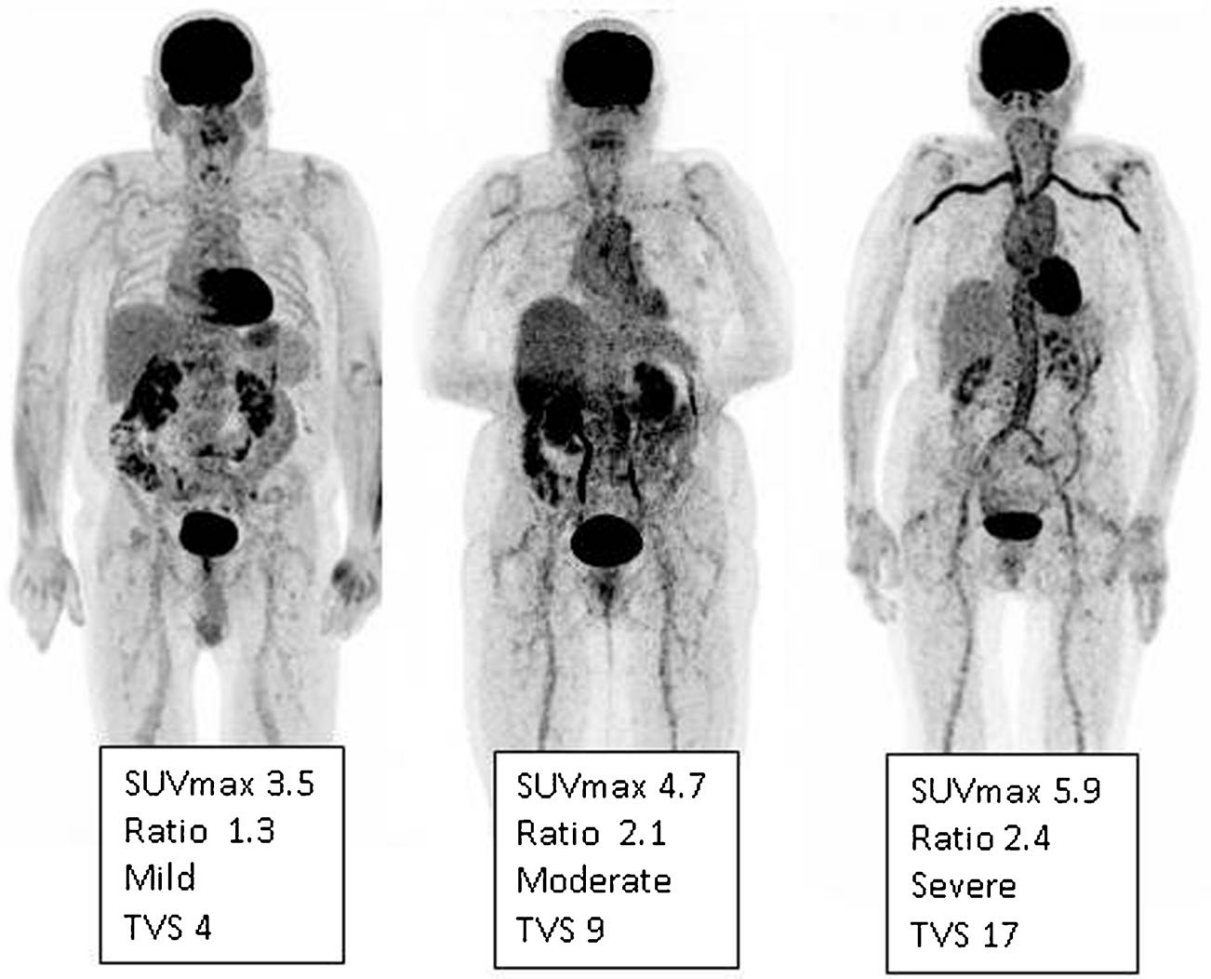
FDG-PET. Low (grade 1), intermediate (grade 2), and high (grade 3) LVV FDG uptake patterns including SUV_max_ values of the thoracic aorta in patients with GCA. Ratio is defined as average SUV_max_ of the thoracic aorta divided by the liver region. The total vascular score (TVS) is the highest for the right-positioned patient
